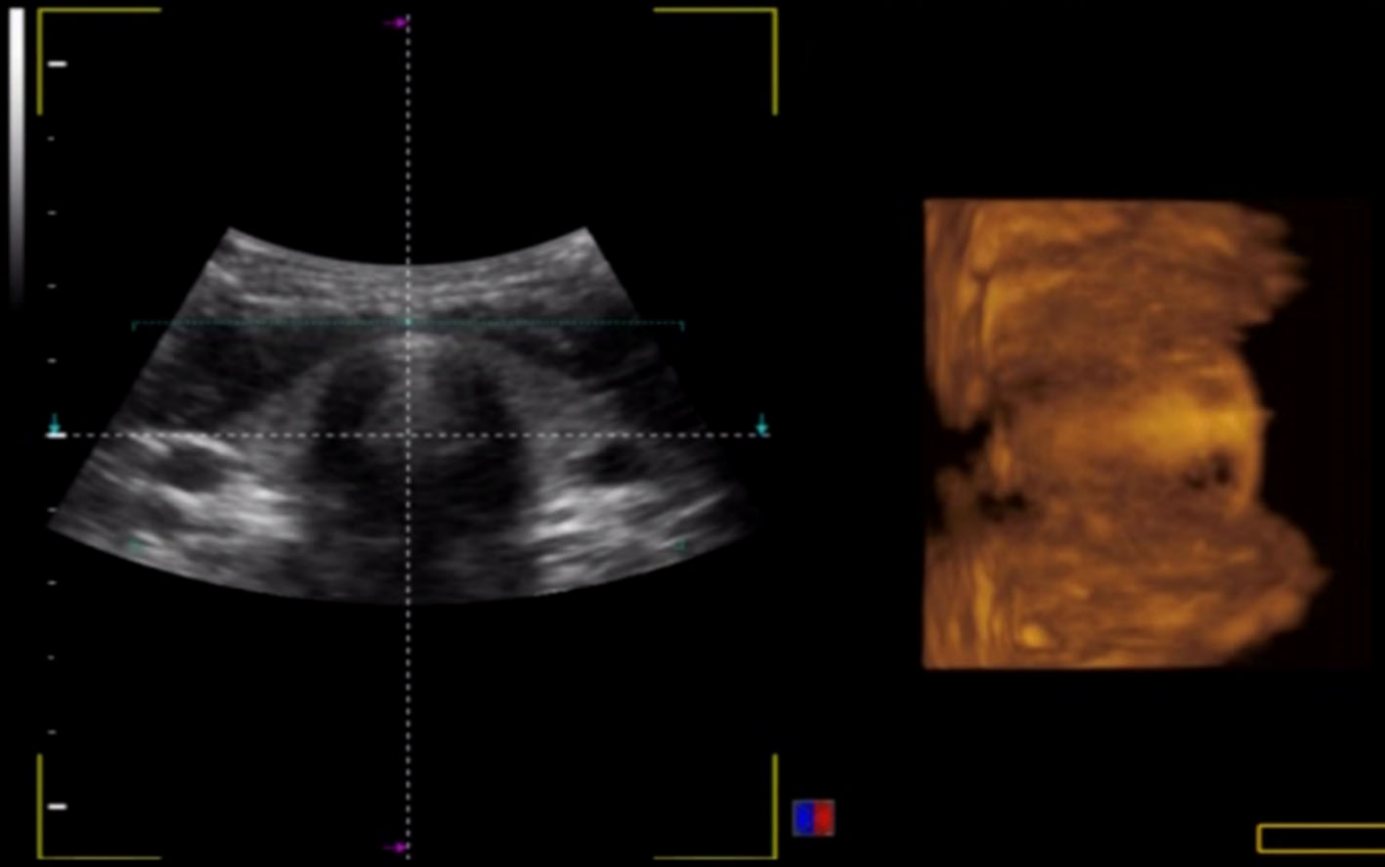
Example of an acquired image by students. Corresponding raw 2D (*left*) and 3D (*right*) ultrasound images were displayed simultaneously. The real-time 3D/4D image is displayed on an orthogonal plane relative to the 2D image
